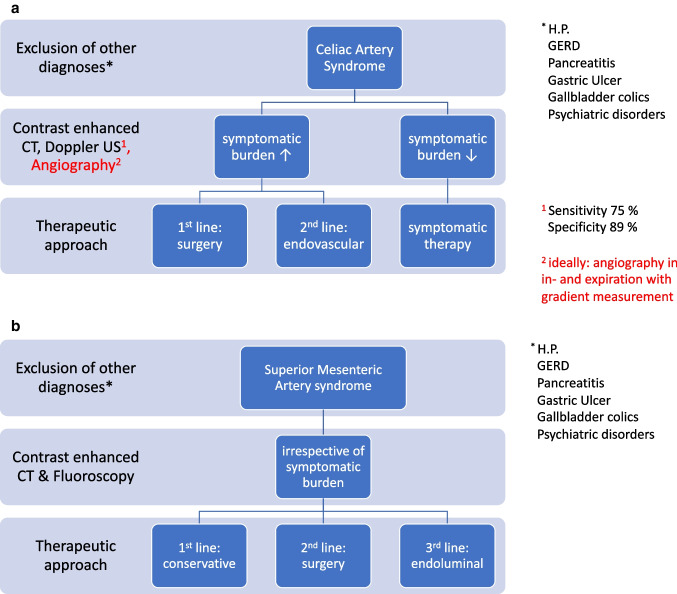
A Celiac artery syndrome work-up and treatment algorithm. B Superior mesenteric artery syndrome work-up and treatment algorithm. HP, Helicobacter pylori; GERD, gastroesophageal reflux disease; US, ultrasound; contrast enhanced computed tomography (CT)
